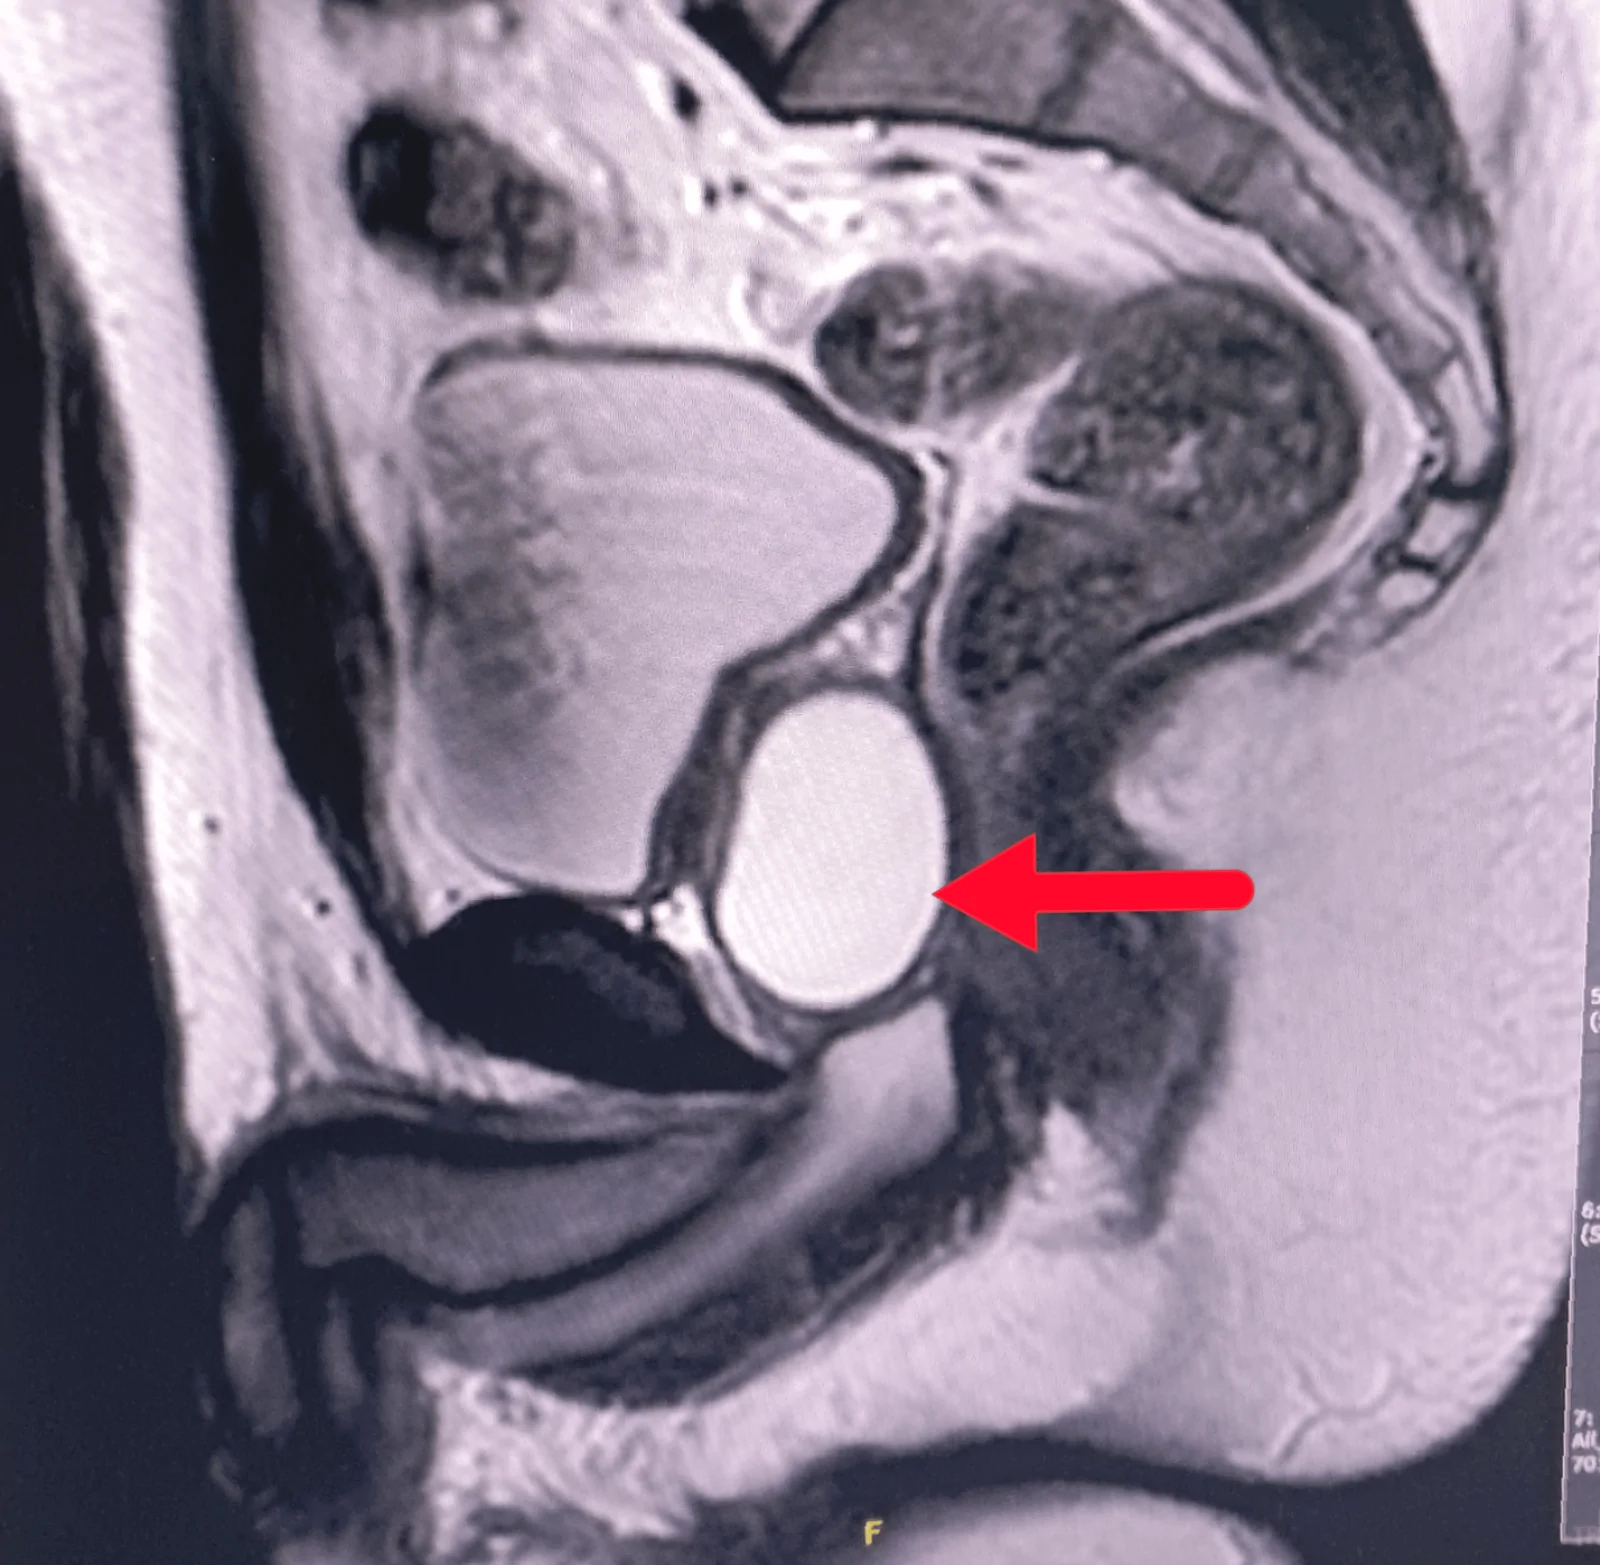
T2 MRI showing a hypeintense left prostatic cyst (red arrow).
